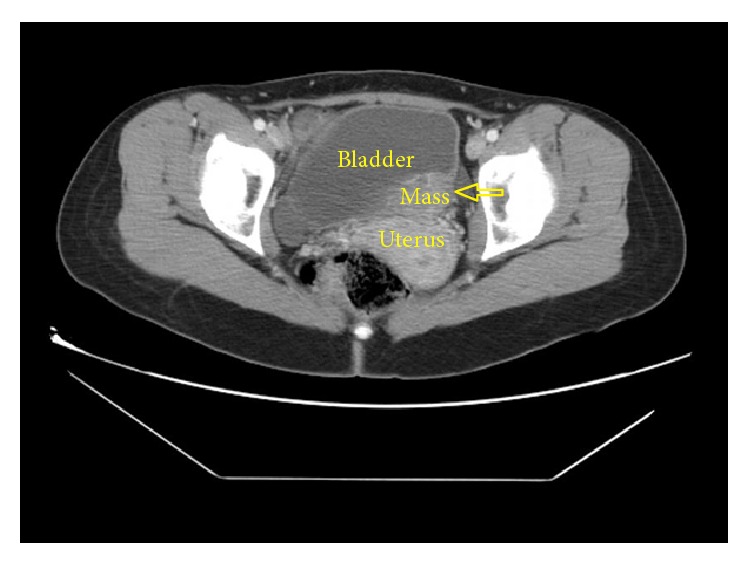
Abdominal contrast Computed Tomography showing a massive lesion of 3 × 4 cm extending into the bladder on the left ureter orifice on the left side wall of the bladder.
